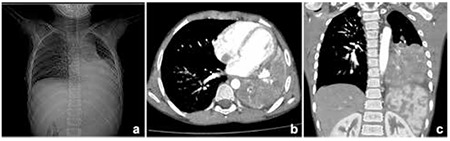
Postero-anterior chest radiography showing a huge area of radioopacity on the left middle and lower hemithorax and calcification in the central area of the opacity (a). Contrast-enhanced arterial phase axial (b) and multiplanar-reformatted coronal (c) chest computed tomography images scan clearly show a giant, calcified soft tissue mass in the left lower hemithorax. Contrast-enhanced axial computed tomography shows heterogeneous enhancement and obscured border between the mass and the heart in some areas, a finding consistent with pericardial involvement
